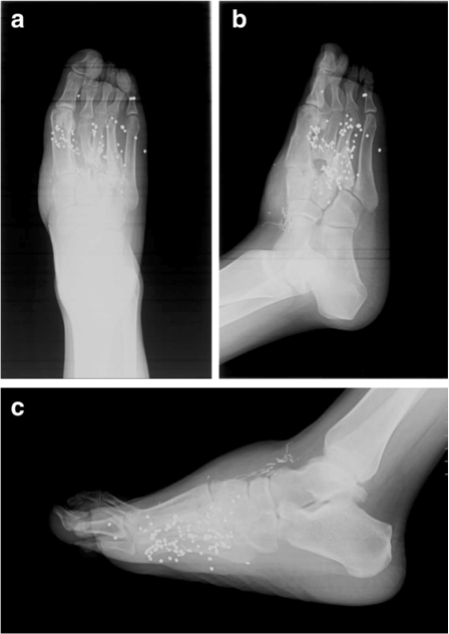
AP (a), oblique (b), and lateral (c) radiographs of the right foot 7 months post-op.
